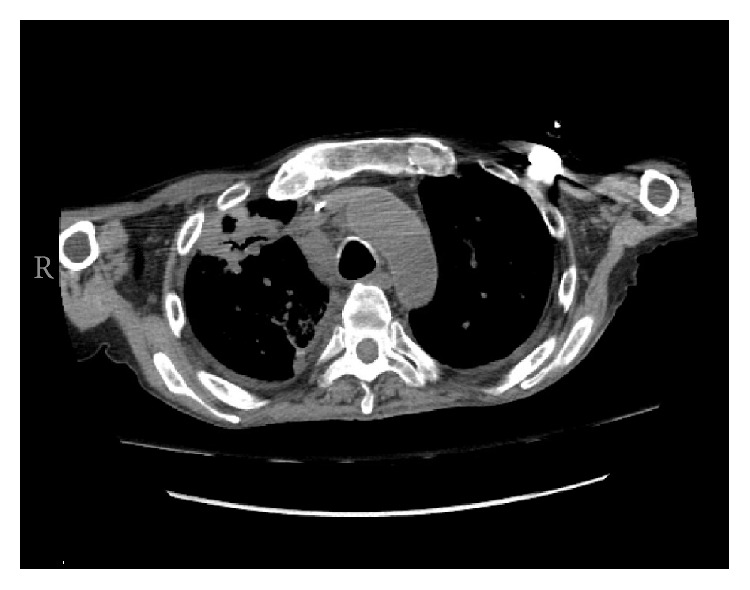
Chest computed tomography scan showing cavitary lesion in the right lung apex.
